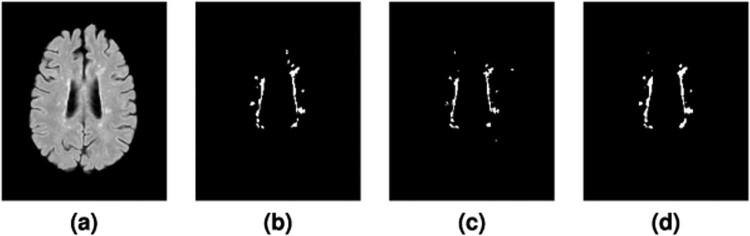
Shown is an axial slice of the (a) FLAIR for a single time-point from one of the Test data set subjects, and the corresponding mask by (b) Rater #1, (c) Rater #2, and the (d) Consensus Delineation.
